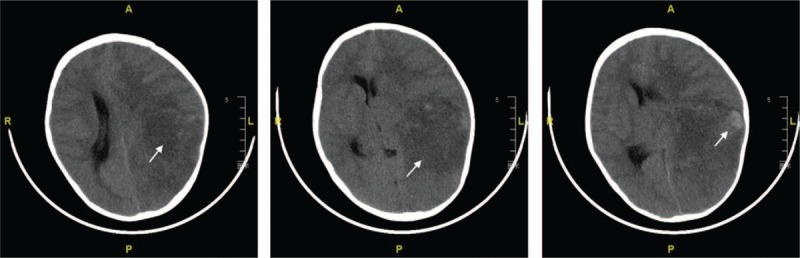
Cranial plain CT scan at the local hospital displayed a large low-density lesion with no clear boundaries in the left frontal, temporal and parietal, part of which was high density (Arrow). The left ventricle was pressed, and the midline shifted to the right.
